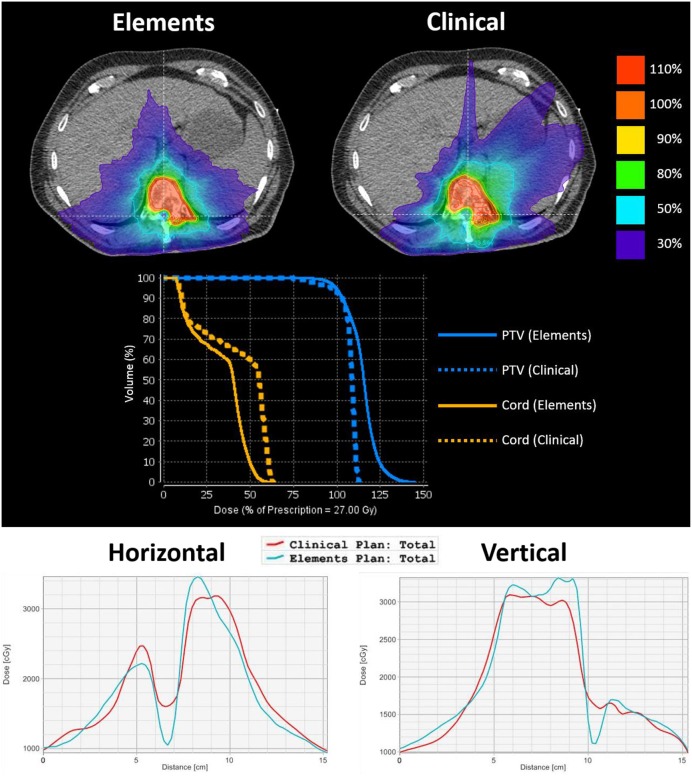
An axial image from case 16's plans to emphasize differences in dose gradient at the spinal cord–target interface. The target volume is delineated by a yellow contour. The top left shows IDLs from the Elements plan, and the top right shows IDLs for the clinical plan. The middle shows the corresponding DVH curves of PTV and spinal cord curves for each plan. The bottom left shows the 2D dose profile along a horizontal line crossing through the cord, and the bottom right shows the 2D dose profile along a vertical line crossing through PTV and spinal cord (lines were shown in the above isodose picture overlaid on axial CT image). From both 2D dose profiles, it can be seen that Elements plan achieved lower cord dose and faster dose fall off between PTV and cord.
